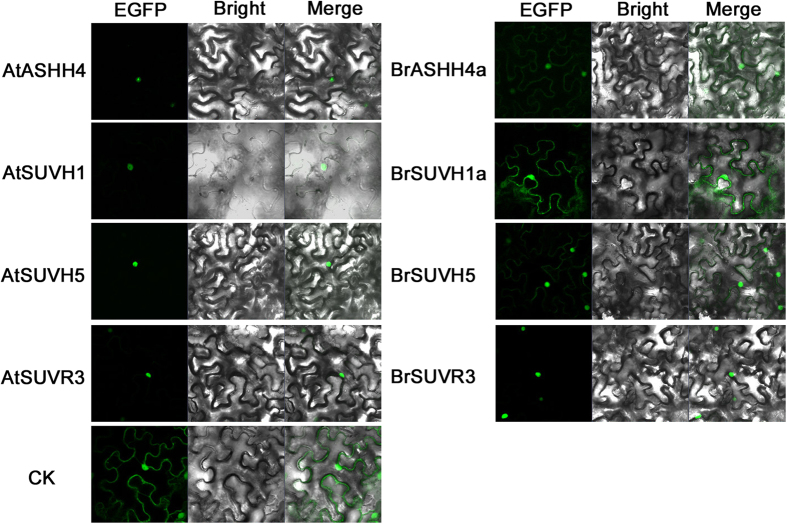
Subcellular localization of homologous *Brassica rapa* and *Arabidopsis thaliana* SDGs in tobacco leaf epidermal cells were transiently expressed using *pFGC-EGFP-SDG* fusion constructs. AtASHH4, AtSUVH1, AtSUVH5, AtSUVR3 and BrSUVR3 are concentrated in the nucleus, whereas BrASHH4a, BrSUVH1a and BrSUVH5 exhibited both cytoplasmic and nuclear localization. *pFGC-EGFP* vector was used as a control (CK).
